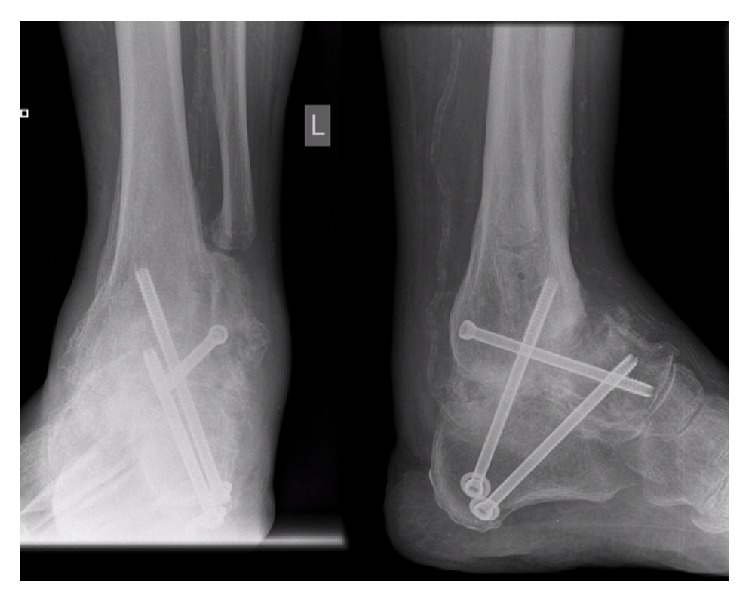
Three-month postsurgery radiographs.
